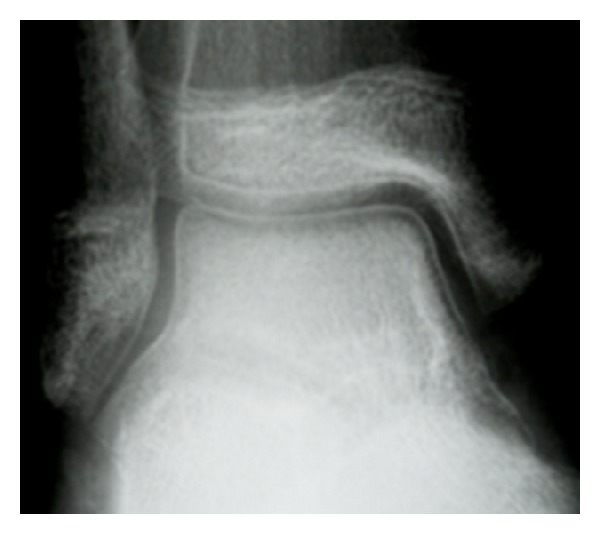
AP-radiograph of the ankle in a 12-year-old girl after immobilization after ankle sprain. Perfect subchondral bandlike osteoporosis of the talar dome can be seen.
